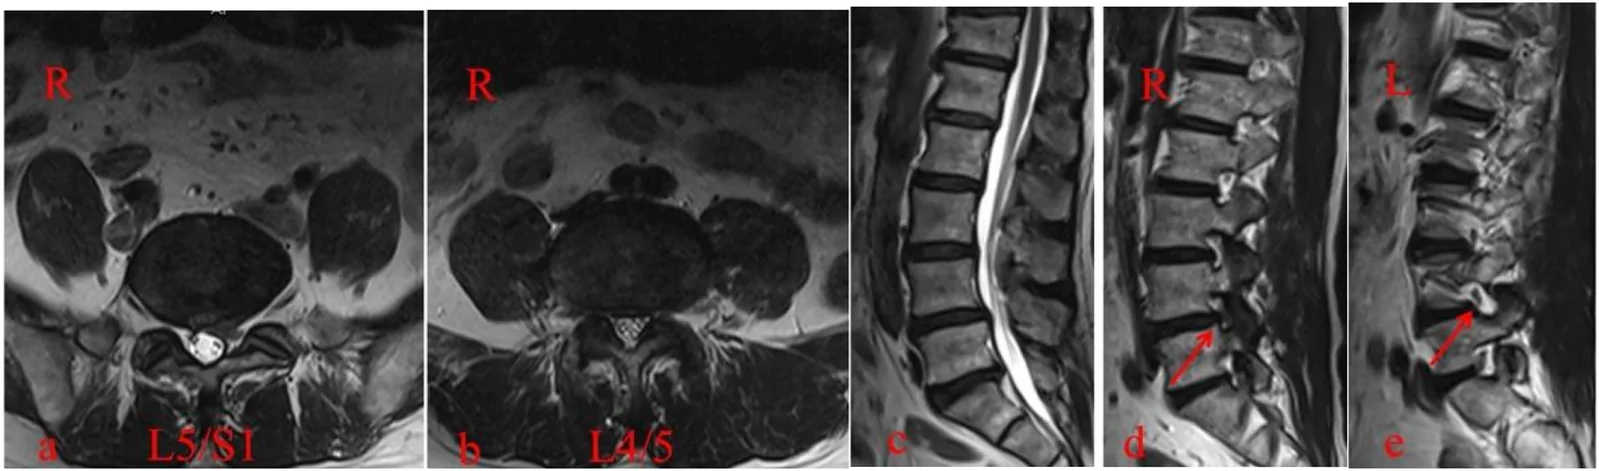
An MRI revealed intervertebral disc herniation at the L5/S1 level and mild central canal and lateral recess stenosis at the L4/5 level (a–c), yet no evident neuroforaminal stenosis was observed on either side (d,e).
